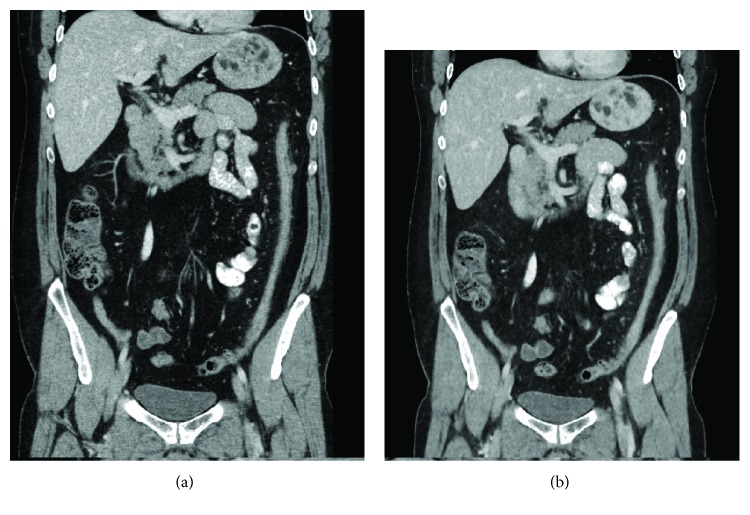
(a) Conventional dose coronal CT image reconstructed with ASIR and (b) low-dose coronal CT image reconstructed with MBIR demonstrating a thickened, featureless descending colon suggestive of chronic inflammation. The low-dose CT entails an approximately 75% radiation dose reduction.
